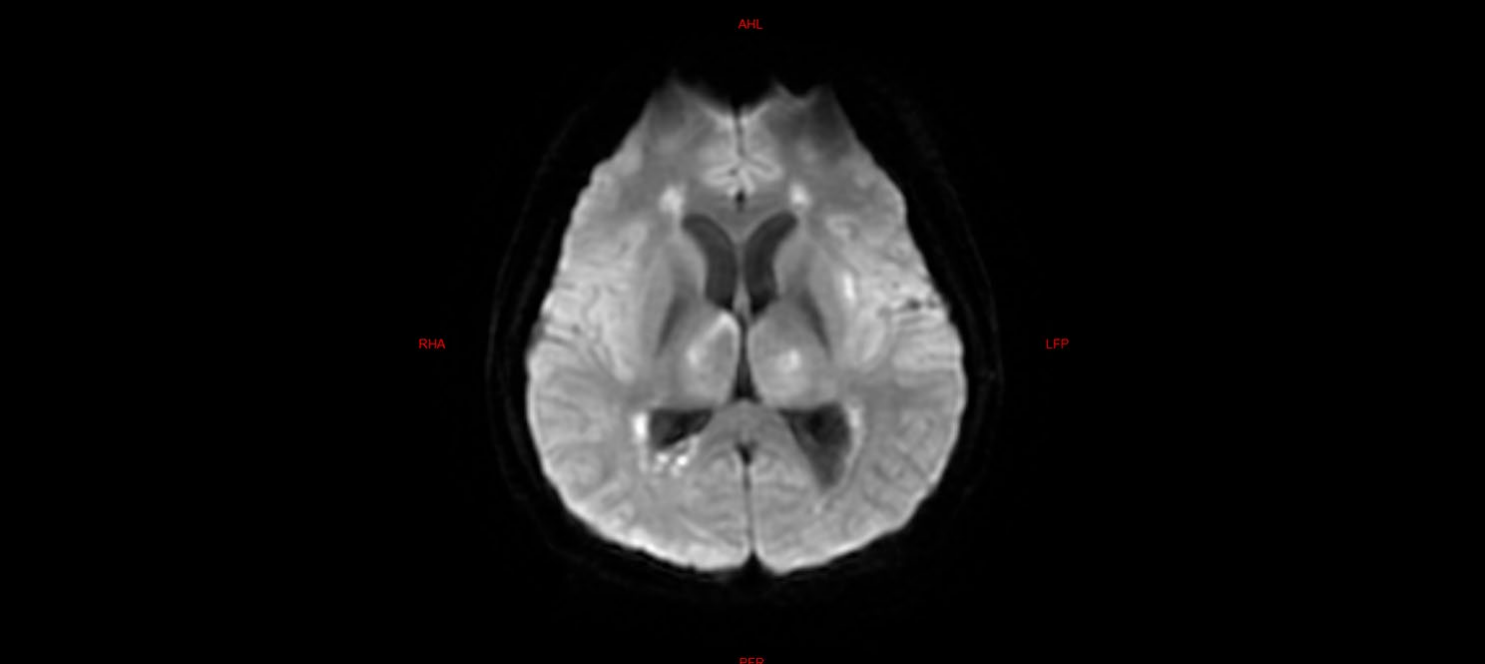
Brain MR DWI sequence of case 9, showed multiple patchy diffuse hyperintensities in the left basal ganglia, bilateral thalamus, and bilateral lateral ventricle white matter. The patient, suspected to have necrotizing encephalopathy, presented in critical condition upon admission, with concurrent multiple organ failure and central diabetes insipidus. Subsequently, due to financial constraints, the family opted to discontinue treatment, leading to the patient’s demise
